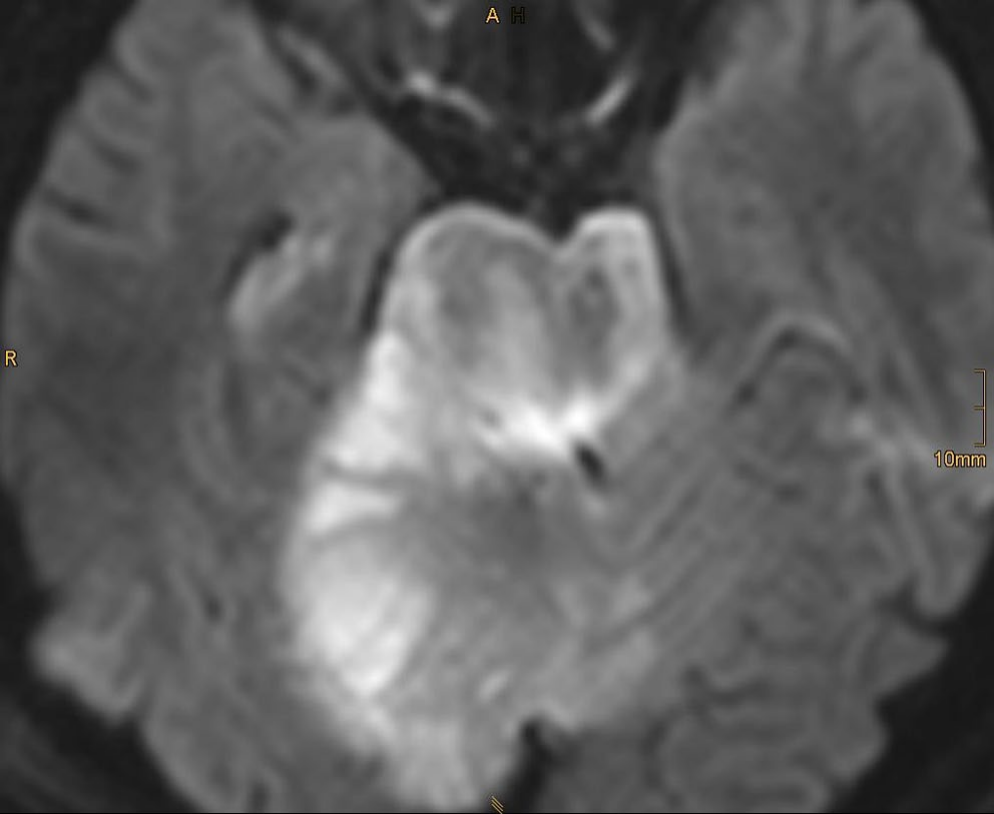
Subsequent magnetic resonance imaging (MRI), however, showed massive brain stem infarction
